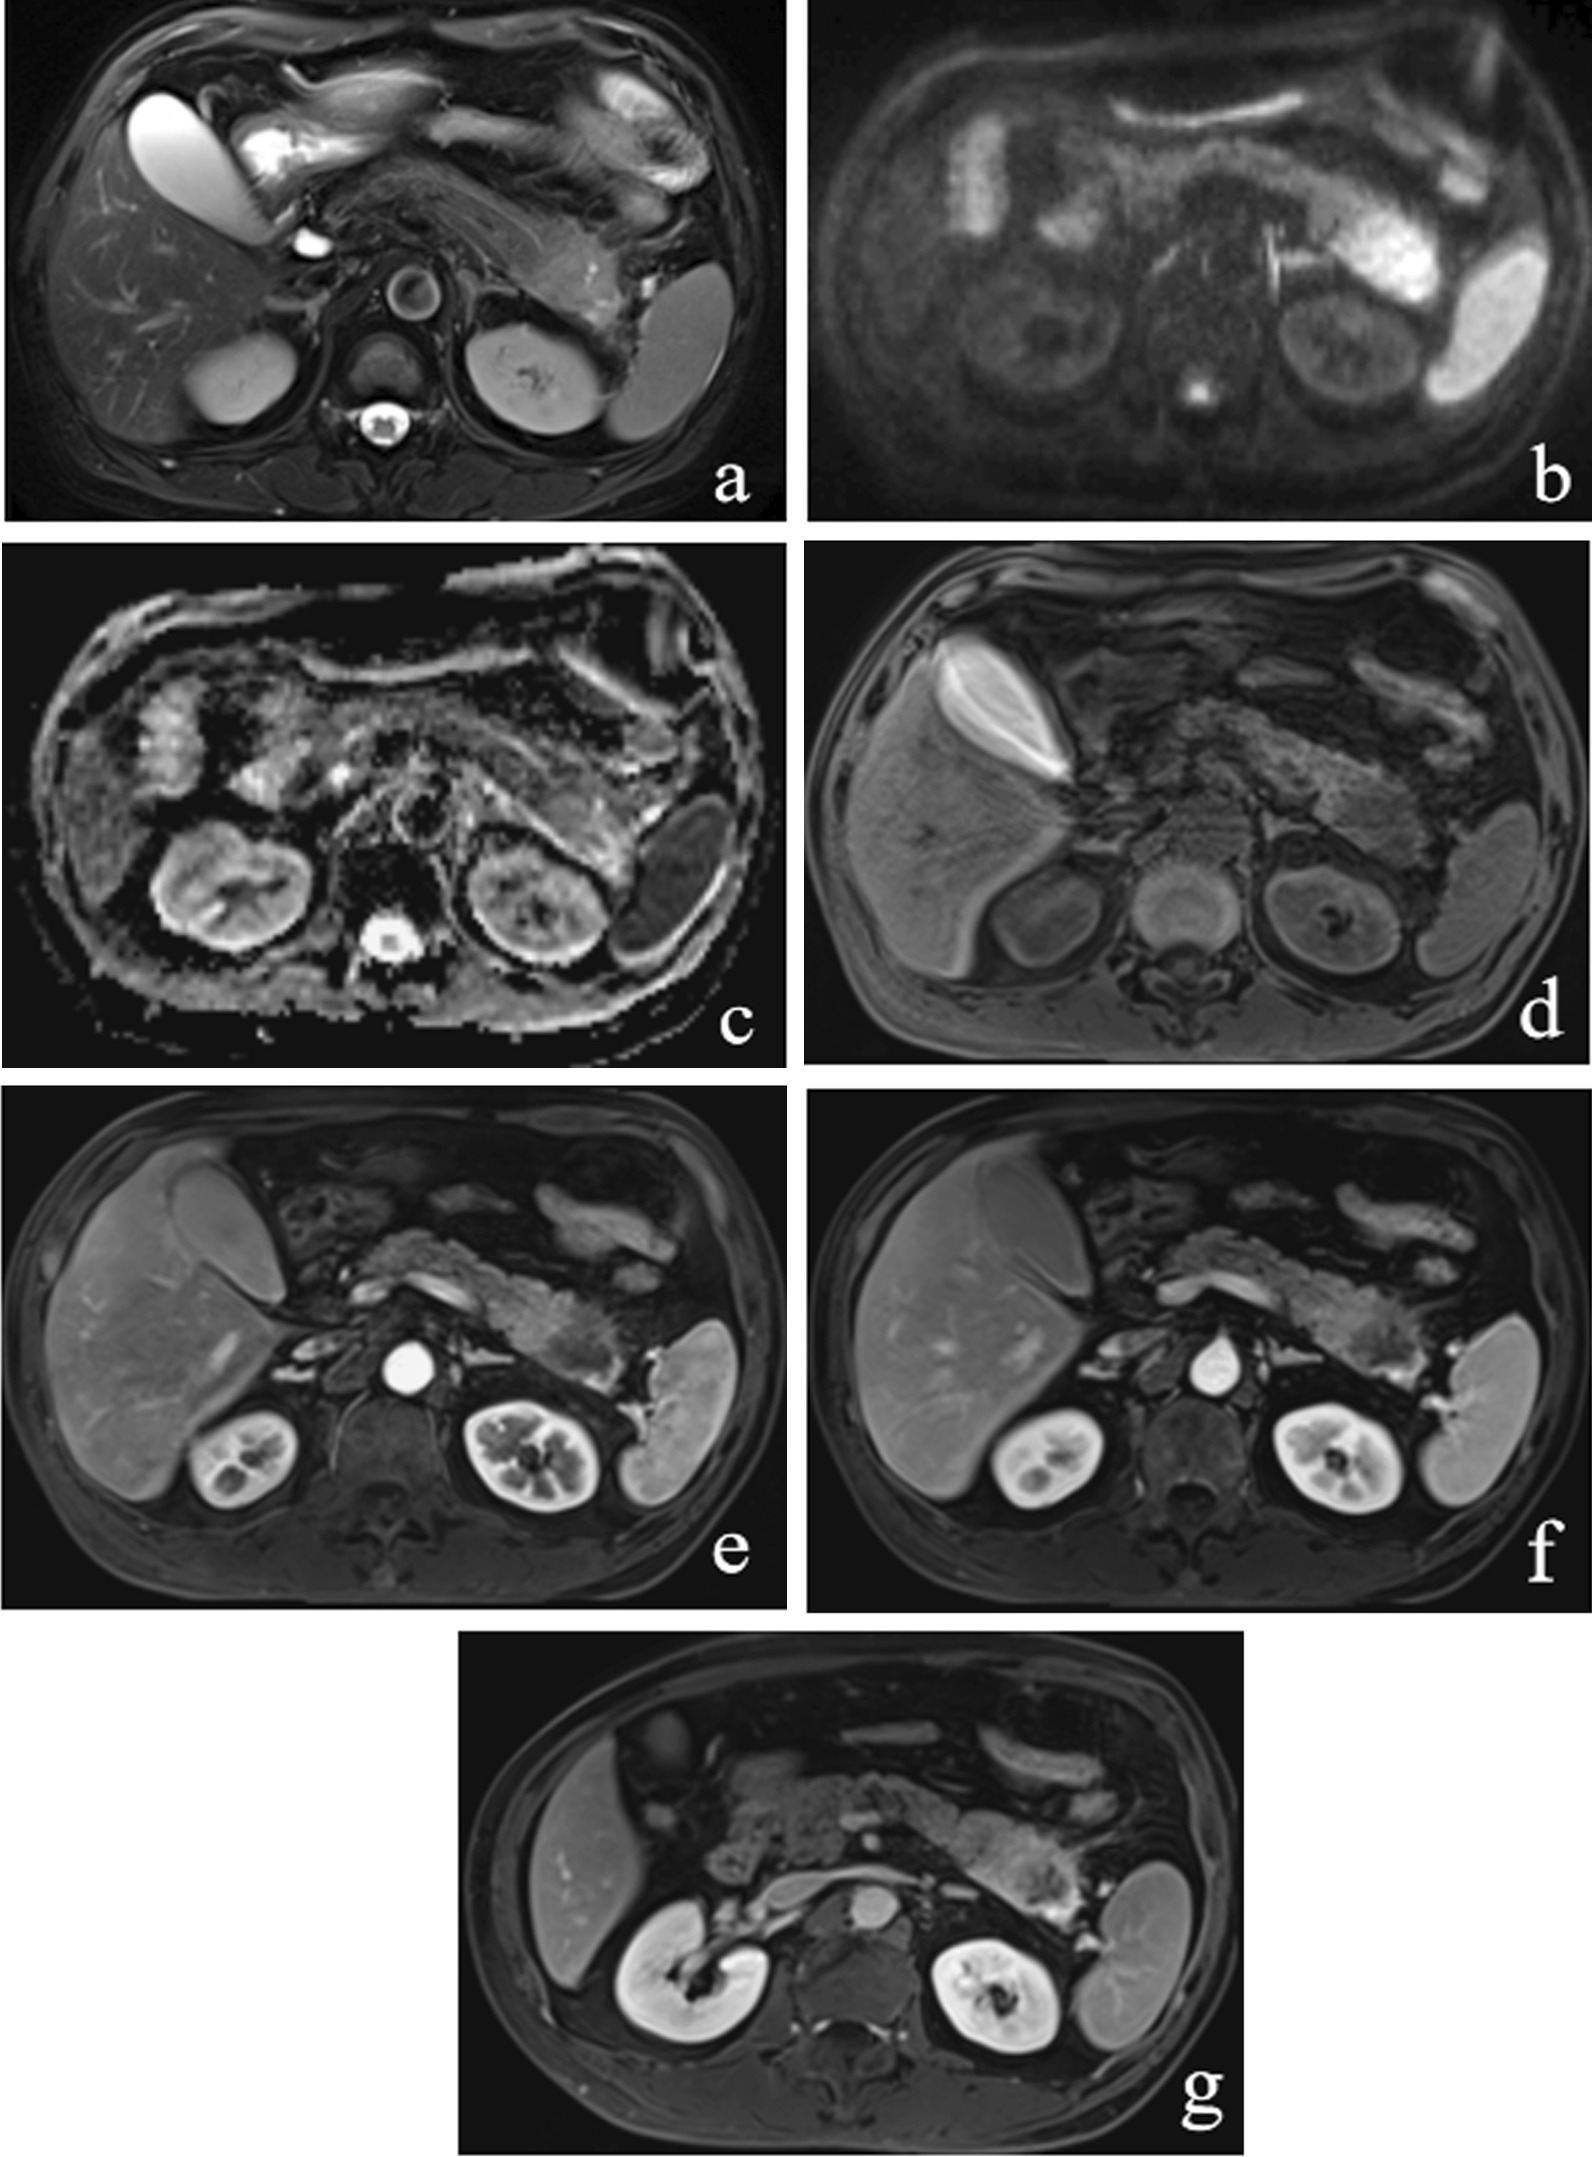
A 62-year-old man had PDAC. a T2WI shows an oval-shaped mass located in the pancreatic tail that is visible as hyperintense with heterogeneity. b On DWI (b = 800 s/mm^2^) and ADC map (c), the pancreatic mass is clearly defined as hyperintense and hypointense, respectively. The pancreatic mass in the tail appears hypointense on both unenhanced (d) and (e) PP images. During the VP (f) and DP (g) imaging, the mass appears mild or obviously hyperintense, but the central area maintains hypointensity all the time
